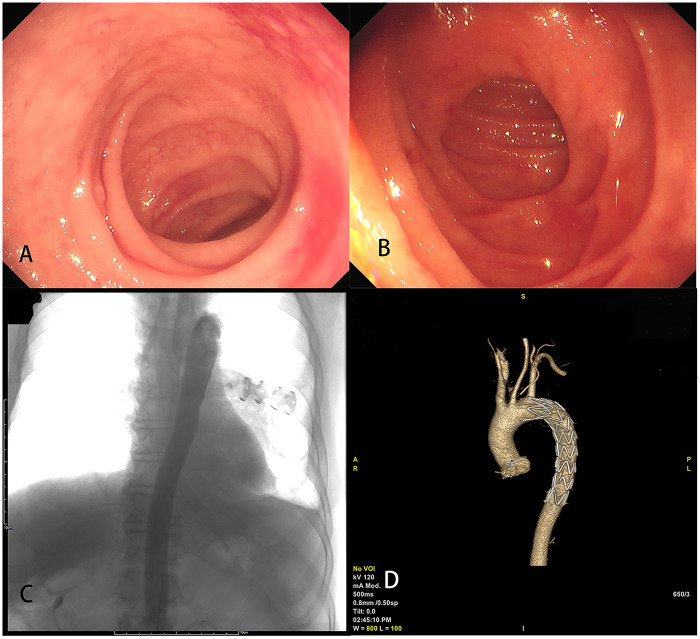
Post-procedural endoscopic and imaging assessment after multidisciplinary management of aortoesophageal fistula. (A) Endoscopic examination at one-month follow-up reveals well-healed esophageal mucosa with no signs of active bleeding or fistula recurrence. (B) Surveillance endoscopy performed six months postoperatively demonstrates sustained mucosal healing without evidence of luminal stenosis or recurrent fistula. (C) Thoracic aortography six months after intervention confirms successful exclusion of the fistula, with no contrast extravasation, indicating complete vascular sealing. (D) Postoperative thoracic computed tomography angiography (CTA) acquired one week following stent-graft deployment shows appropriate positioning and fixation of the endovascular prosthesis, with no endoleak or residual hemorrhage.
